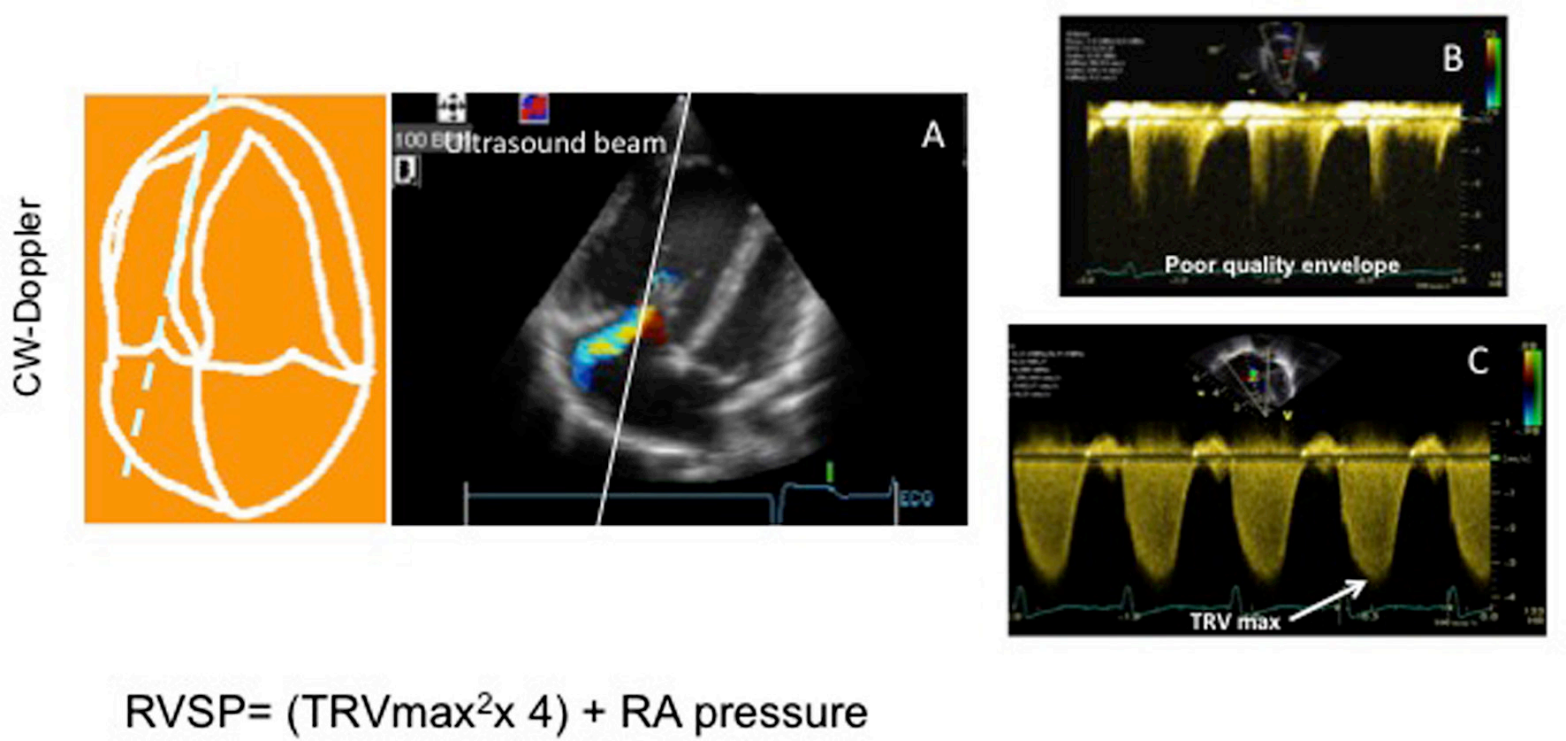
Tricuspid regurgitant velocity in a patient with pulmonary hypertension. (A) Four-chamber view showing a tricuspid jet the aligned ultrasound beam for assessment of tricuspid velocity. (B) Image showing a poor quality envelop of the tricuspid jet that does not allow accurate measurement. (C) Good tricuspid envelope allowing measurement of the maximal tricuspid regurgitant jet velocity (TRV_max_).
